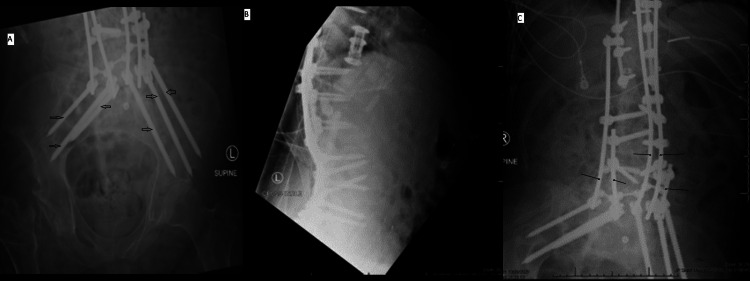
Postoperative radiographs following the second operation The pelvic fracture and lumbopelvic dissociation were corrected by adding another rod to create a five-rod construct and increasing the number of pelvic fixation points to six, up from four previously. A. Anteroposterior radiograph of the pelvis with each point of fixation denoted (arrows). B. Lateral radiograph of the pelvis. C. Anteroposterior radiograph of the lumbar spine and superior pelvis, with all five rods in the construct denoted (lines).
